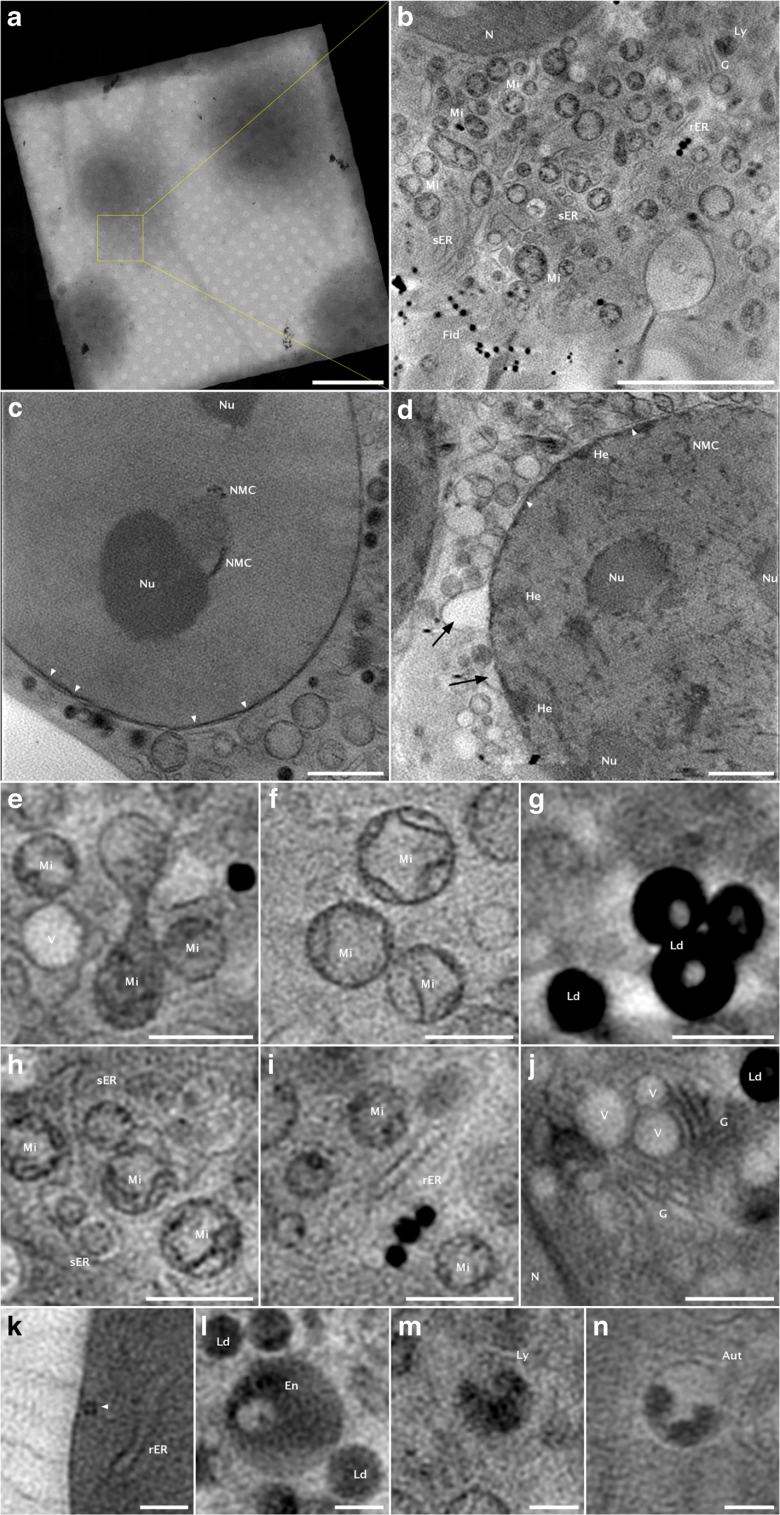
An overview of cryo soft X-ray data of mammalian cells (fibroblast and NIH-3T3 cells). The different structures have been labeled as follows: N, nucleus; NMC, nuclear membrane channel; Nu, nucleolus; Mi, mitochondria; He, heterochromatin; G, Golgi apparatus; rER, rough endoplasmic reticulum; sER, smooth endoplasmic reticulum; Fid, fiducial markers; Ld, lipid droplets; V, vesicles; Ly, lysosome; En, endosome; Aut, autophagosome. a A mosaic overview of a square within the grid with a cell of interest. Within the mosaic, areas for acquiring tilt-series are selected. The yellow box represents the field of view of the camera. b One slice of the reconstructed volume from the yellow box shown in a. c, d One slice of a reconstructed volume showing the N of two different cells. In c, two Nu are visible, as well as some NMC. The white arrows aim at pores within the double nuclear membrane. In d, Nu is visible and He structures can be seen close to the nuclear membrane. The white arrowheads point to the double membrane. The black arrows point to a detachment of the outer layer of the nuclear membrane, which is called nuclear blebbing. e,f Two Mi forms we were able to observe. A small and elongated form (e) and some swollen Mi (f). g The two forms of Ld usually appear as filled or with an empty core. h, i ER can be found between other organelles. While rER is elongated and usually easy to find, sER membranes are thinner and appear to be randomly distributed. j The G is usually surrounded by low absorbing V and appears as parallel oriented elongated structures close to the N. k, l, m, n Endocytic vesicles in different stages. Depending of the developmental and metabolic state of the cell, different forms can be found. Scale bars: a 20 μm; b 5 μ; c, d 2 μm; e, f, g, h, i, j 1 μm; k, l, m, n 0.5 μm
